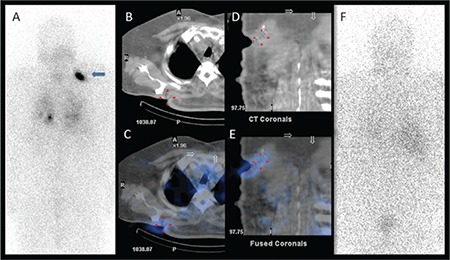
An intense I-131 uptake was identified at the upper zone of the right hemithorax (arrow) on posterior image of post-therapeutic I-131 whole body scan (A) in addition to lung metastasis. On axial computed tomography (CT) (B) and fused single photon emission computed tomography (SPECT)/CT images (C), the finding was adjacent to the right scapula, and localized to the skin/subcutaneous soft tissue. In addition, on coronal CT (D) and SPECT/CT images (E) surgical sutures related to a non-thyroidal soft tissue excision prior to radioiodine treatment were detected. On follow-up I-131 scan (F) there wasn’t any pathologic uptake but the patient still had a mildly elevated serum thyroglobulin level (7.5 ng/mL) with thyroid-stimulating hormone stimulation
